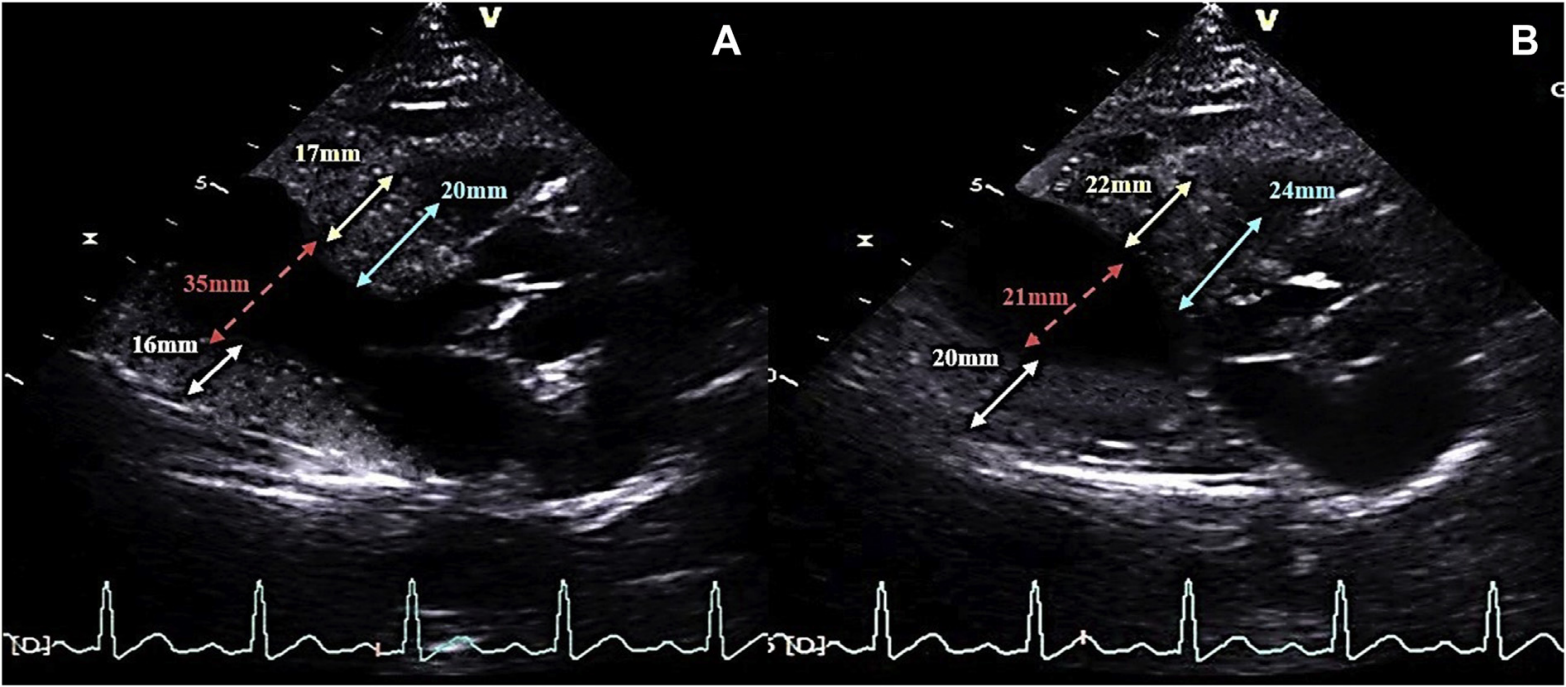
(A) Two-dimensional transthoracic echocardiogram (TTE) parasternal long-axis view in diastole showing dimensions of the interventricular septum (17 mm, *yellow arrows*), ventricular cavity (35 mm, *red arrows*), posterior wall (16 mm, *white arrows*), and basal septum (20 mm, *blue arrows*). (B) Two-dimensional TTE of parasternal long-axis view in systole showing the dimensions of the interventricular septum (22 mm), ventricular cavity (21 mm), posterior wall (20 mm), and basal septum (24 mm).
